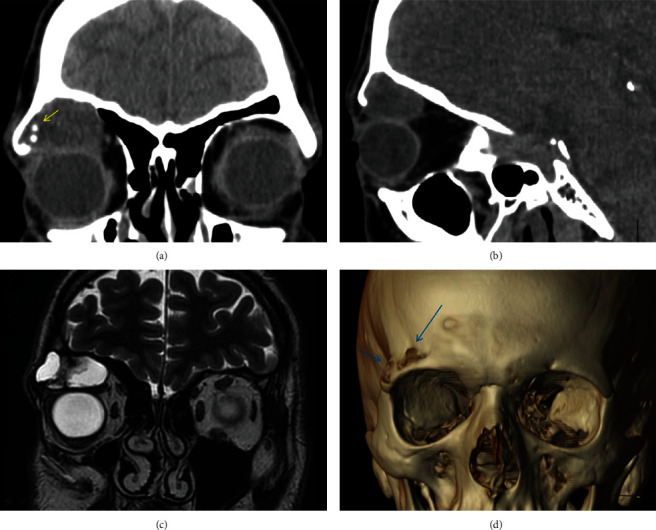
(a, b) Coronal and sagittal CT images, demonstrating an expansive lesion arising from the roof of the right orbit with soft tissue attenuation. Small foci of mineralization (arrow) into the lesion are presented. The lesion protrudes into the orbit. (c) Coronal T2-weighted MR image shows a well-defined lesion arising from the bone with an extraosseous component. The majority of the tumor has high signal intensity on the T2-weighted image, with low signal areas which represent the solid component of the tumor. (d) Volume-rendered 3D-CT reconstruction images show lytic areas with bone destruction in the roof of the orbit.
